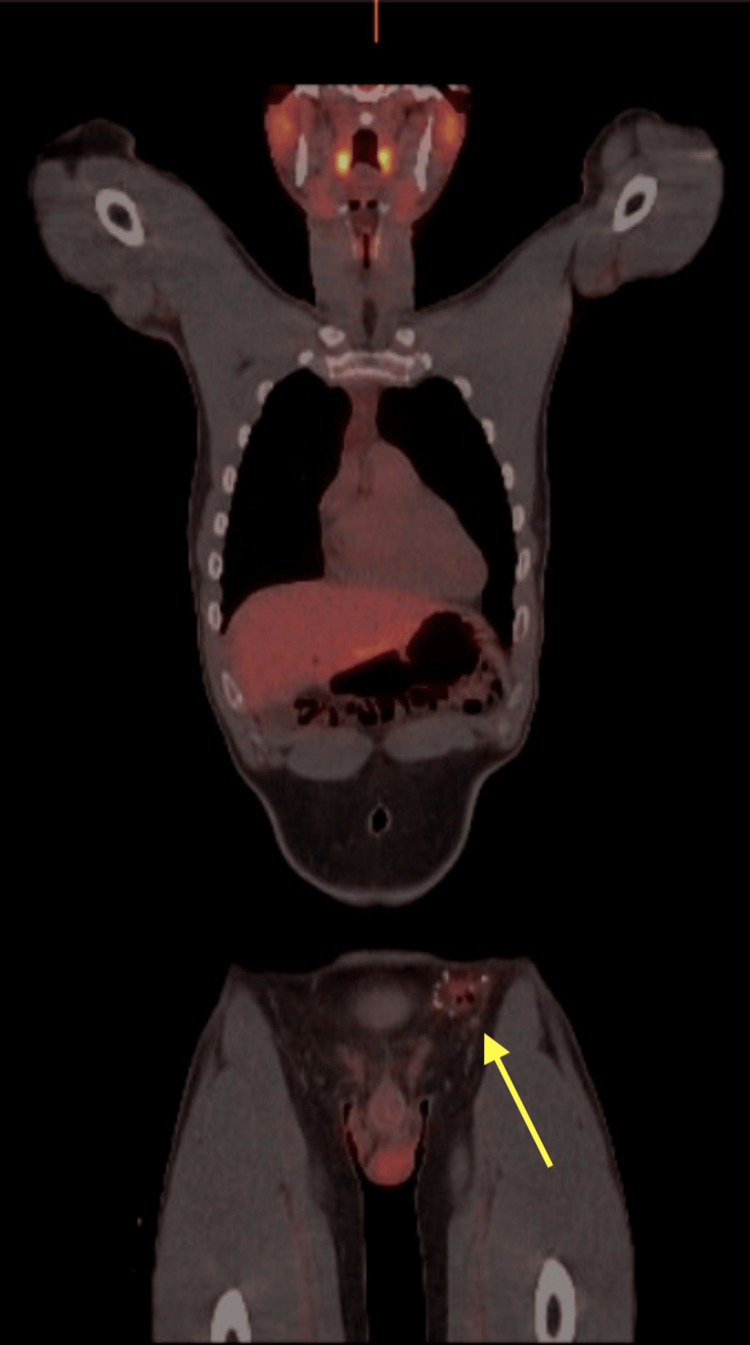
PET of the skull base to mid-thigh There is mild increased FDG uptake within the anterior left groin region consistent with postoperative inflammatory changes which is indicated by the yellow arrow in the figure. No additional abnormal FDG uptake in the neck, chest, abdomen, or pelvis was seen, and there were no osseous lesions identified PET: positron emission tomography; FDG: fludeoxyglucose F18
